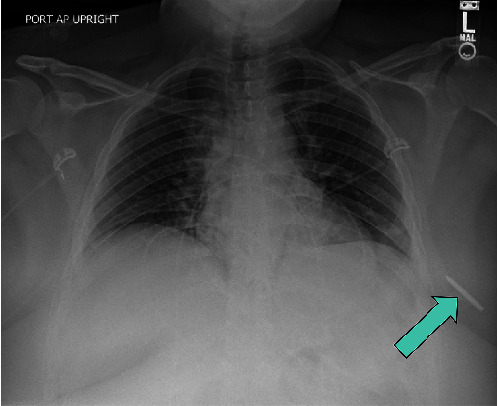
Chest X-ray demonstrating the foreign body in the left breast.
